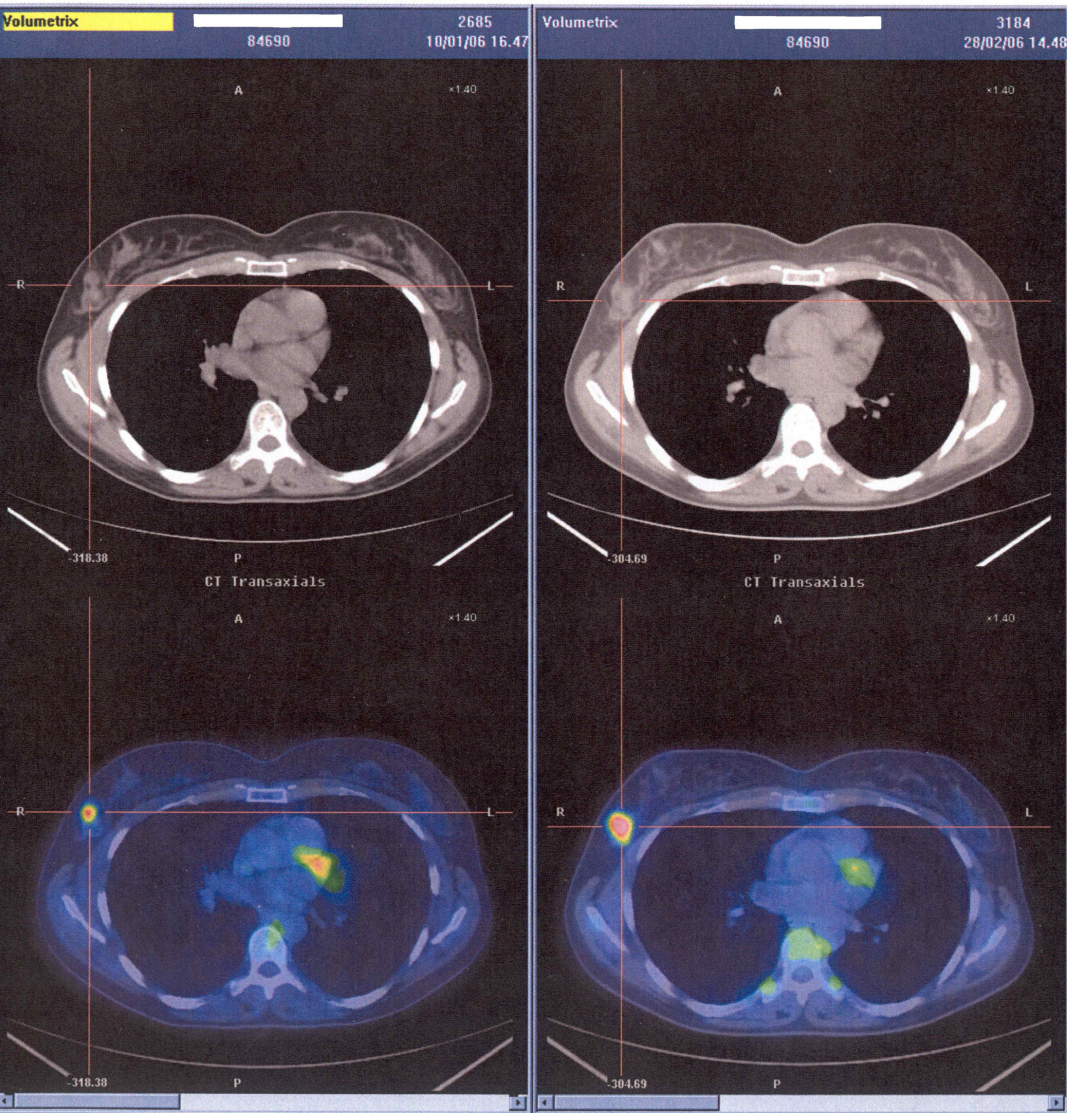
A PET-CT shows a local relapse of disease.
